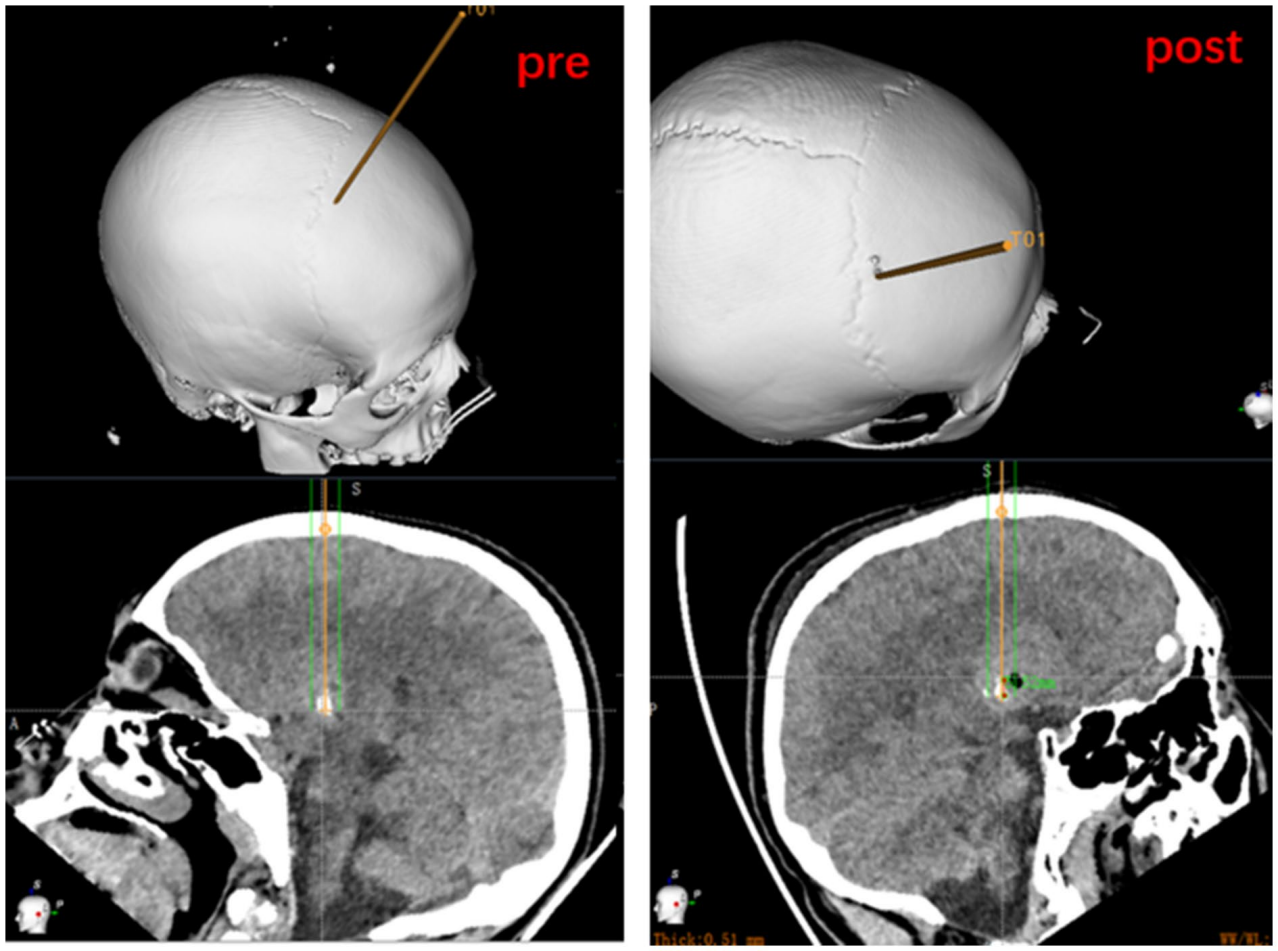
Measurement of entry point and target point error based on preoperatively planned target and on the fusion of postoperative CT to the preoperative dataset.
